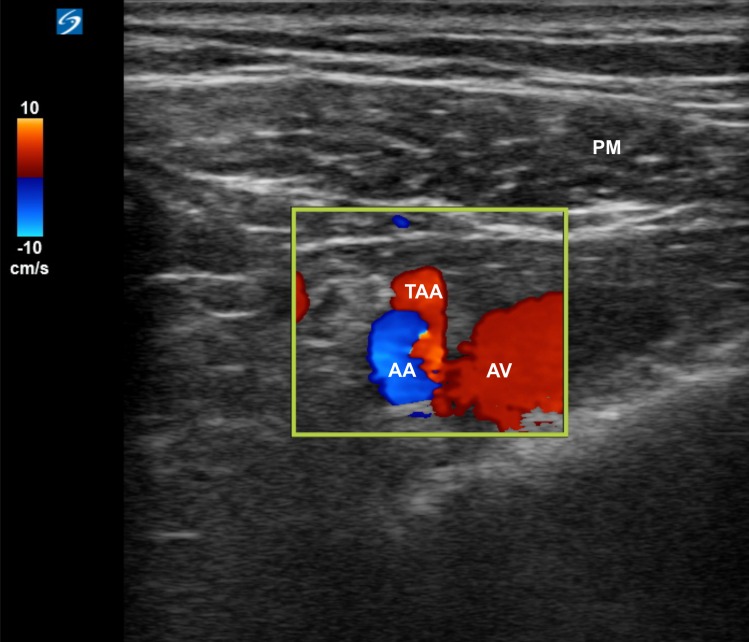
Ultrasound image of the thoracoacromial artery originating from the axillary artery. AA: axillary artery, AV: axillary vein, TAA: thoracoacromial artery, PM: pectoralis major muscle.
